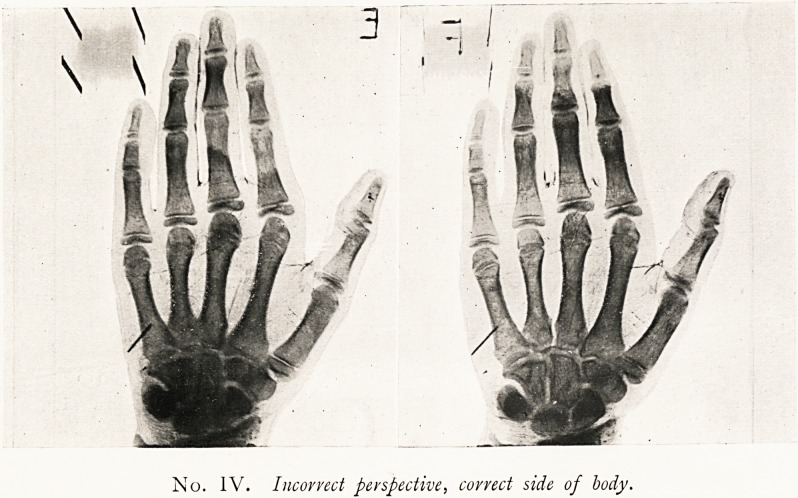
# Stereoscopic X-Ray Representation, with an Example
